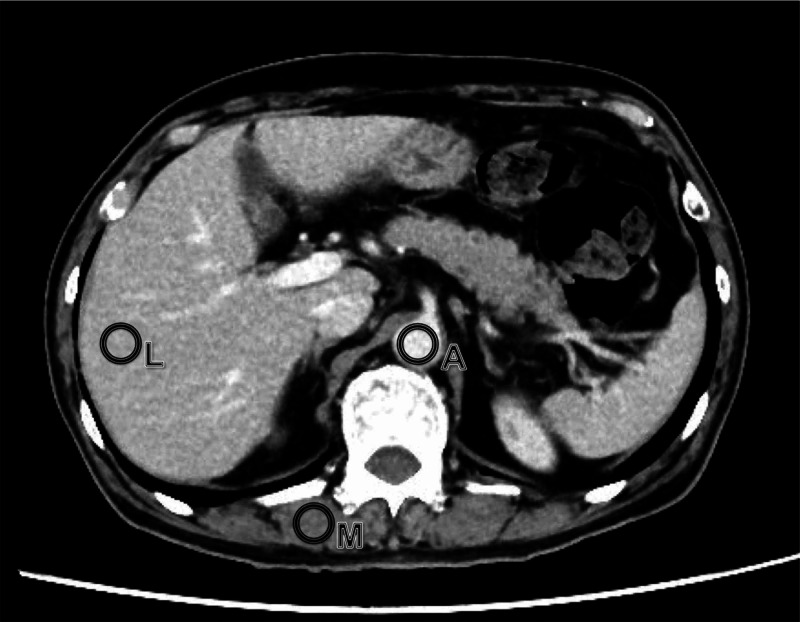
CT scan of a 69-year-old man with bladder cancer This figure is an axial image of the beginning of the celiac artery in the 80-kV FIRST protocol. The ROIs were placed in aorta (A), liver (L), and the erector spinae muscle (M). CT, computed tomography; FIRST, forward-projected model-based iterative reconstruction solution; ROI, region of interest
